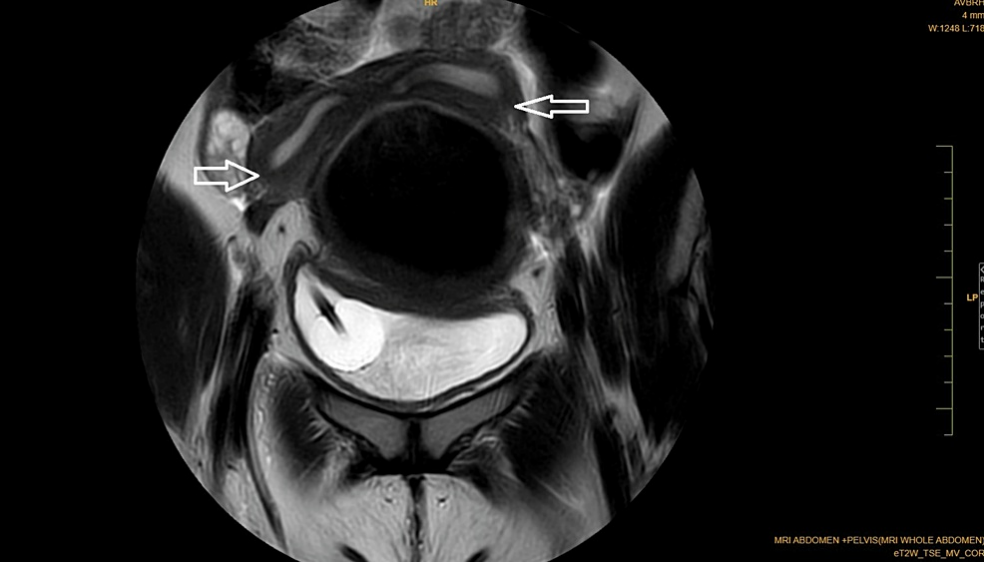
Coronal T2WI showing two separated divergent uterine horns (white arrows) with fundal cleft suggestive of uterine didelphys with hematocolpos. The urinary bladder shows a Foley bulb in situ. Note: Authors' original image T2WI: T2-weighted image
